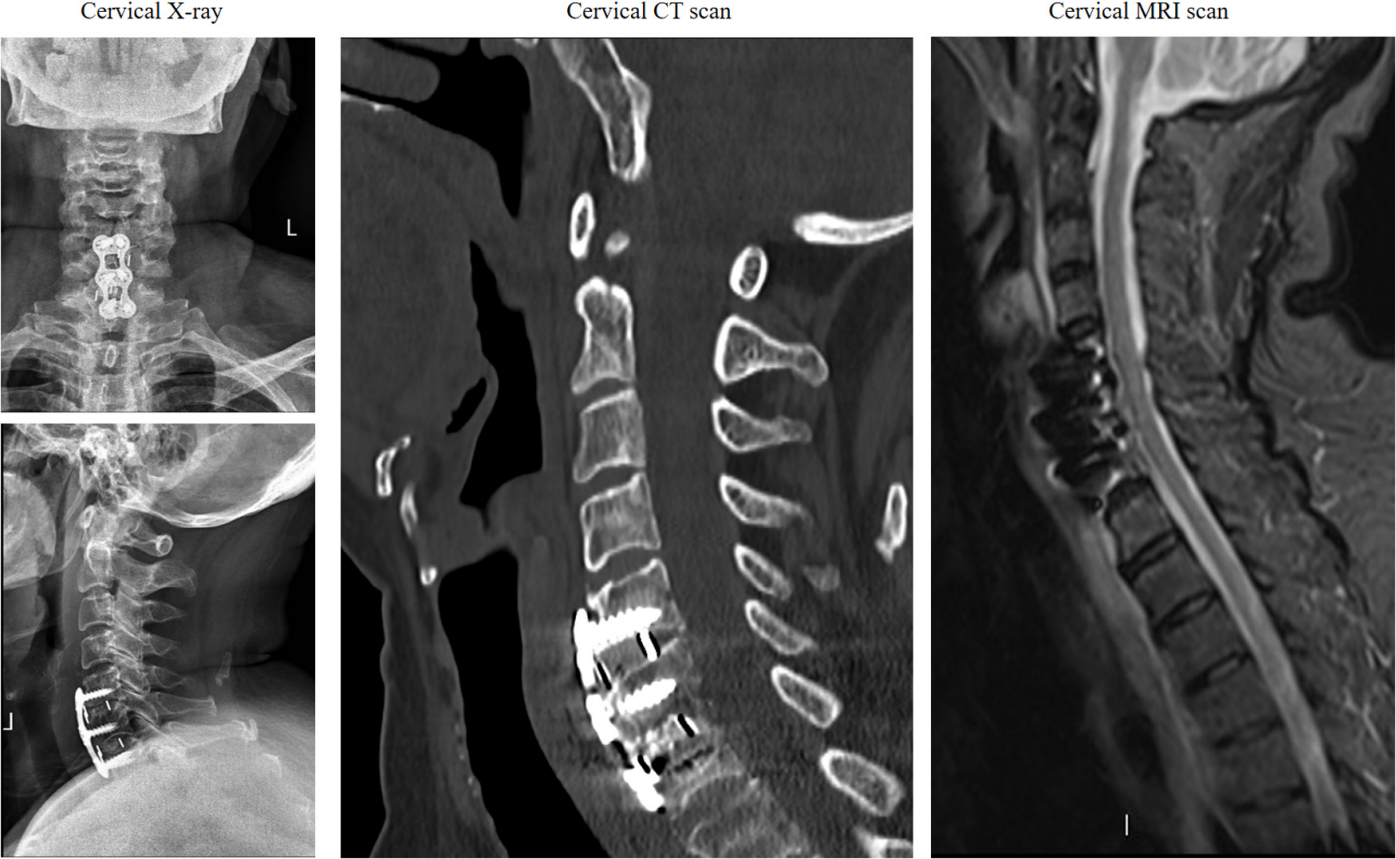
Postoperative cervical spine x-ray and CT imaging.
